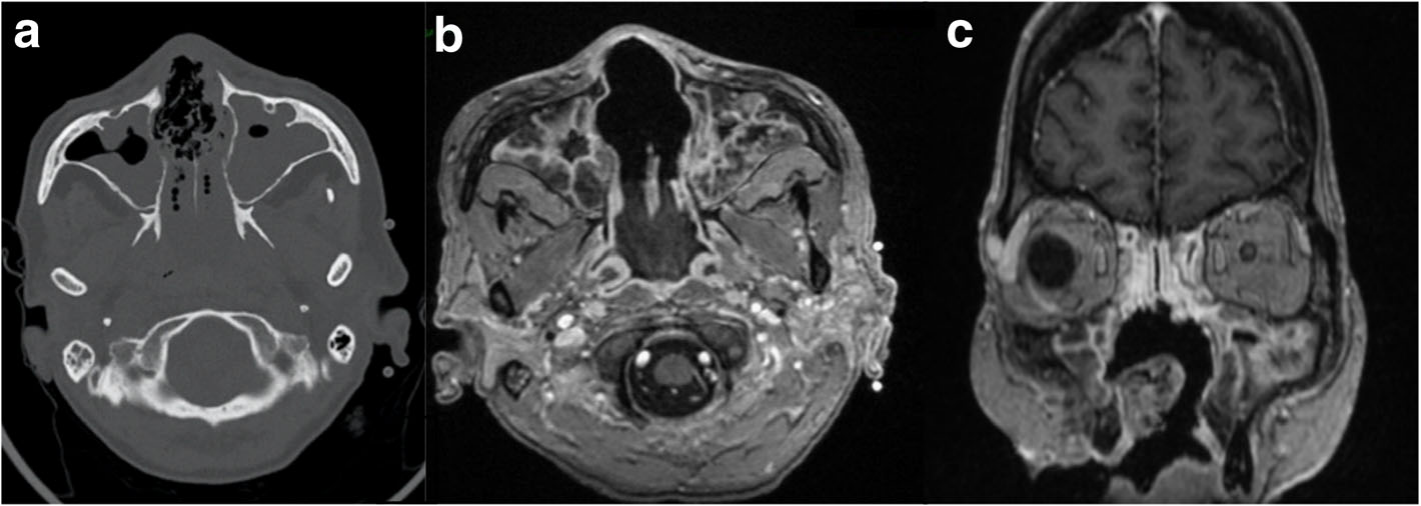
Imaging exploration. a: Sinus CT-scan showing bony nasal septum lysis and bilateral maxillary sinus opacities; b and c: Gadolinium-enhanced T1 weighted MRI (b: axial section; c: frontal section) showing bony nasal septum lysis and maxillar lysis without enhanced tumor mass
